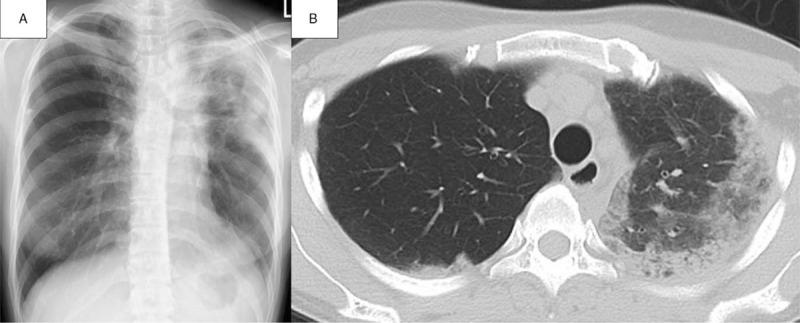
Radiography and chest high-resolution computed tomography (CT) scans. A: Pulmonary infiltrates in the left upper lobe on chest radiography. B: Bilateral peripheral consolidation and ground-glass opacity in the left upper lobe on chest CT.
